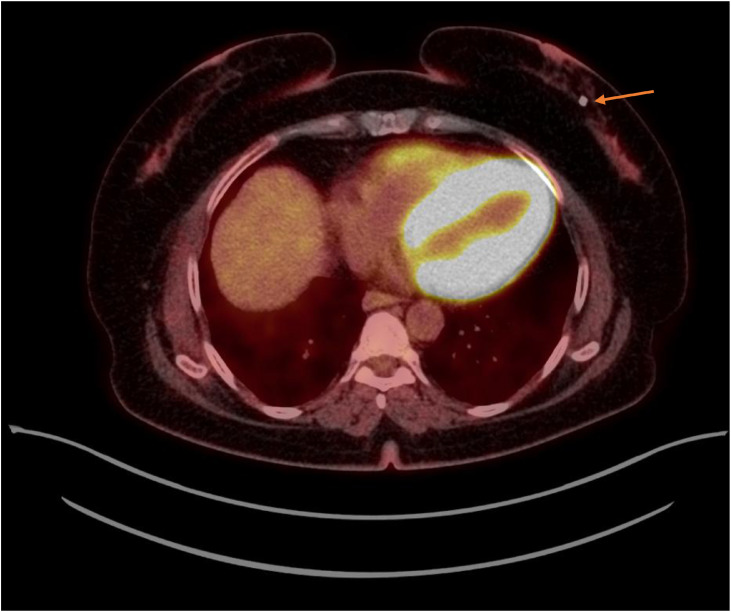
Follow-up whole-body 18F-FDG PET scan showed no evidence of hypermetabolic nodal or breast lymphomatous disease recurrence, with the arrow indicating the left breast marker.
